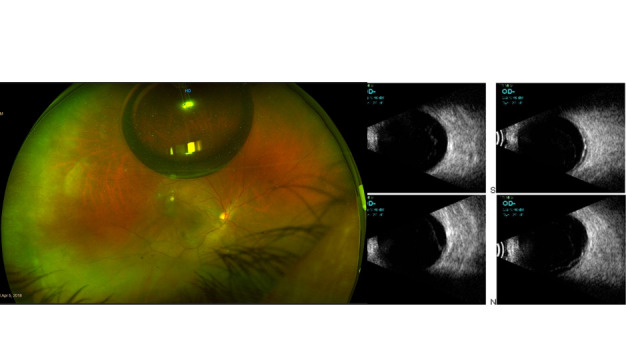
A Optos wide-field fundus photography showed inferior RD with laser scars temporal to the fovea with a small residual gas bubble; B Ultrasound B scan showed shallow retinal detachment
